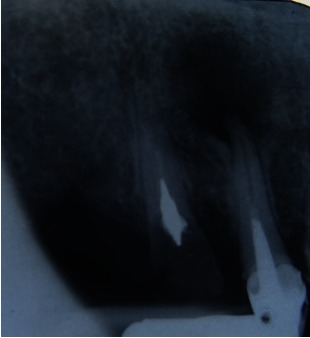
Fig. 112 preoperative radiograph
